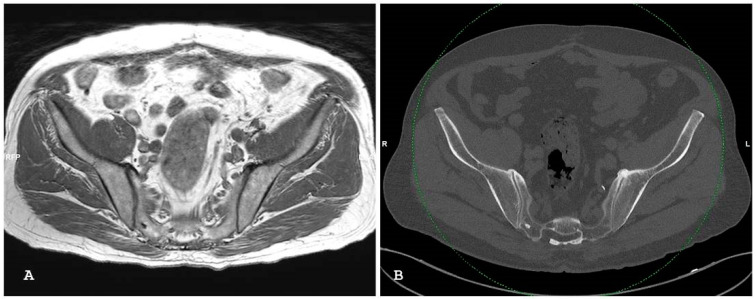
Oblique coronal T1-weighted magnetic resonance imaging sequence (A) shows entheseal bridging anterior to the sacroiliac joints. Axial computed tomography with bone window (B) shows bony bridging anterior to the sacroiliac joints. There are no subchondral erosions or sclerosis.
